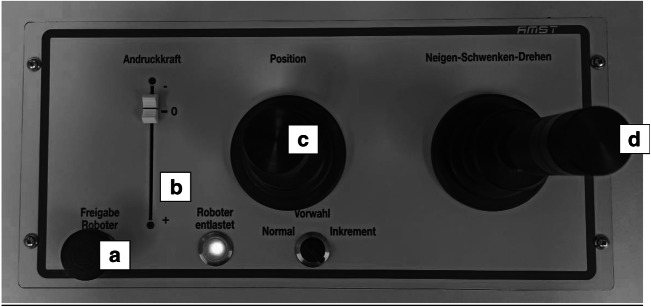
Remote control panel for the robotic ultrasound system. a Safety button b Slide control to adjust probe pressure on subject’s skin. Maximal applicable pressure with the slide control can be limited in the software between 1 and 100 N) c 2-dimensional linear axes joystick d 3-dimensional rotatory control
